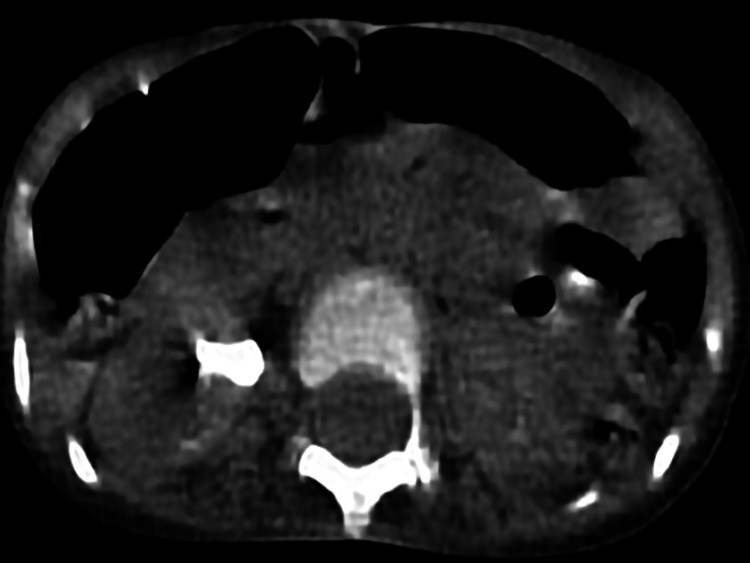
Preoperative CT scan showing a partial staghorn stone along with medullary nephrocalcinosis.
